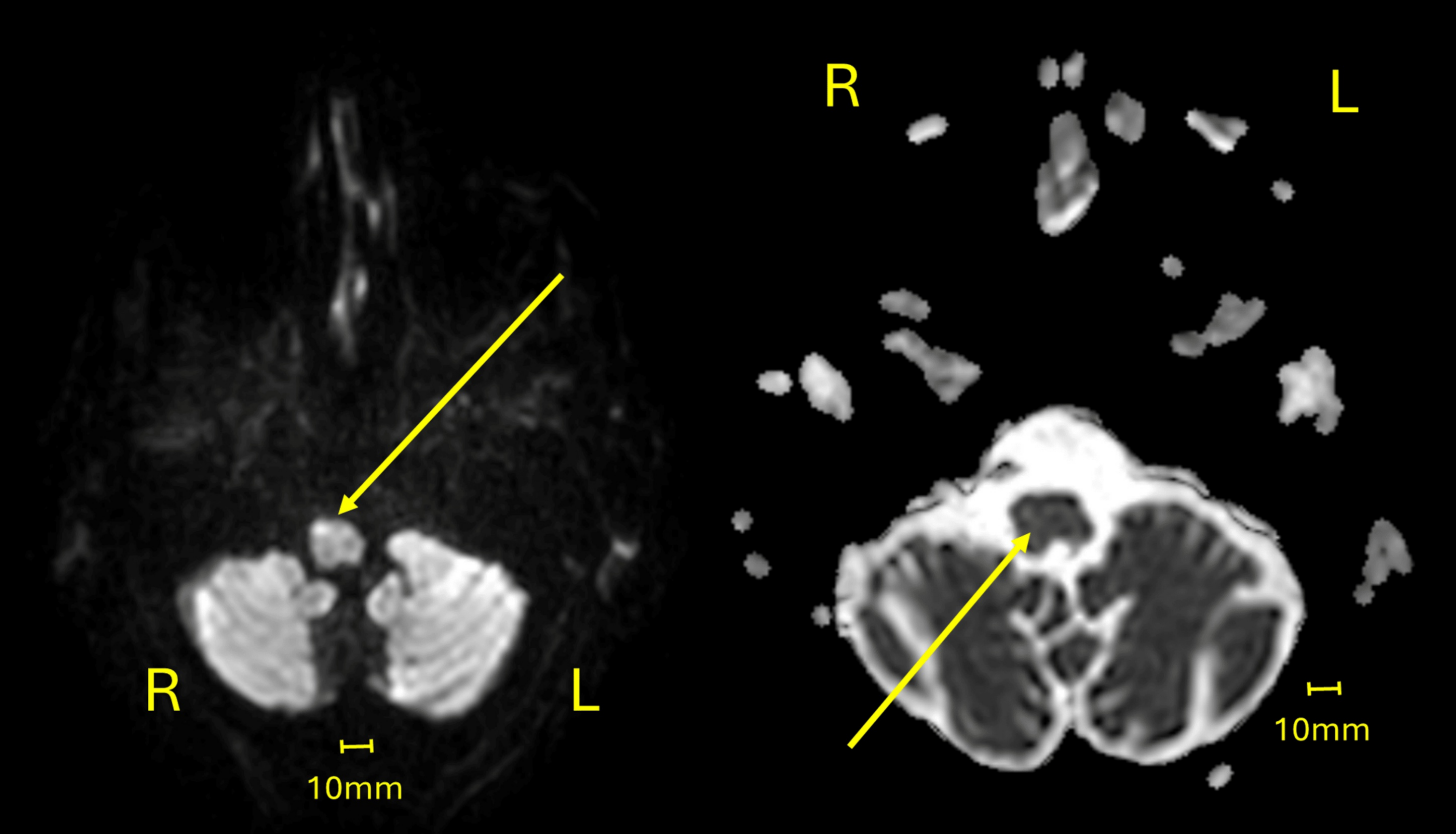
Axial DWI and ADC maps used to exclude ischemic changes Left image: DWI axial view demonstrating a subtle increase in signal intensity within the ION (arrow). Right image: ADC axial view showing no corresponding change in signal intensity in the same region (arrow). Note: Due to the nature of DWI, DWI and ADC images inherently have lower resolution and clarity, but they remain diagnostically reliable. ADC, apparent diffusion coefficient; DWI, diffusion-weighted imaging; ION, inferior olivary nucleus
